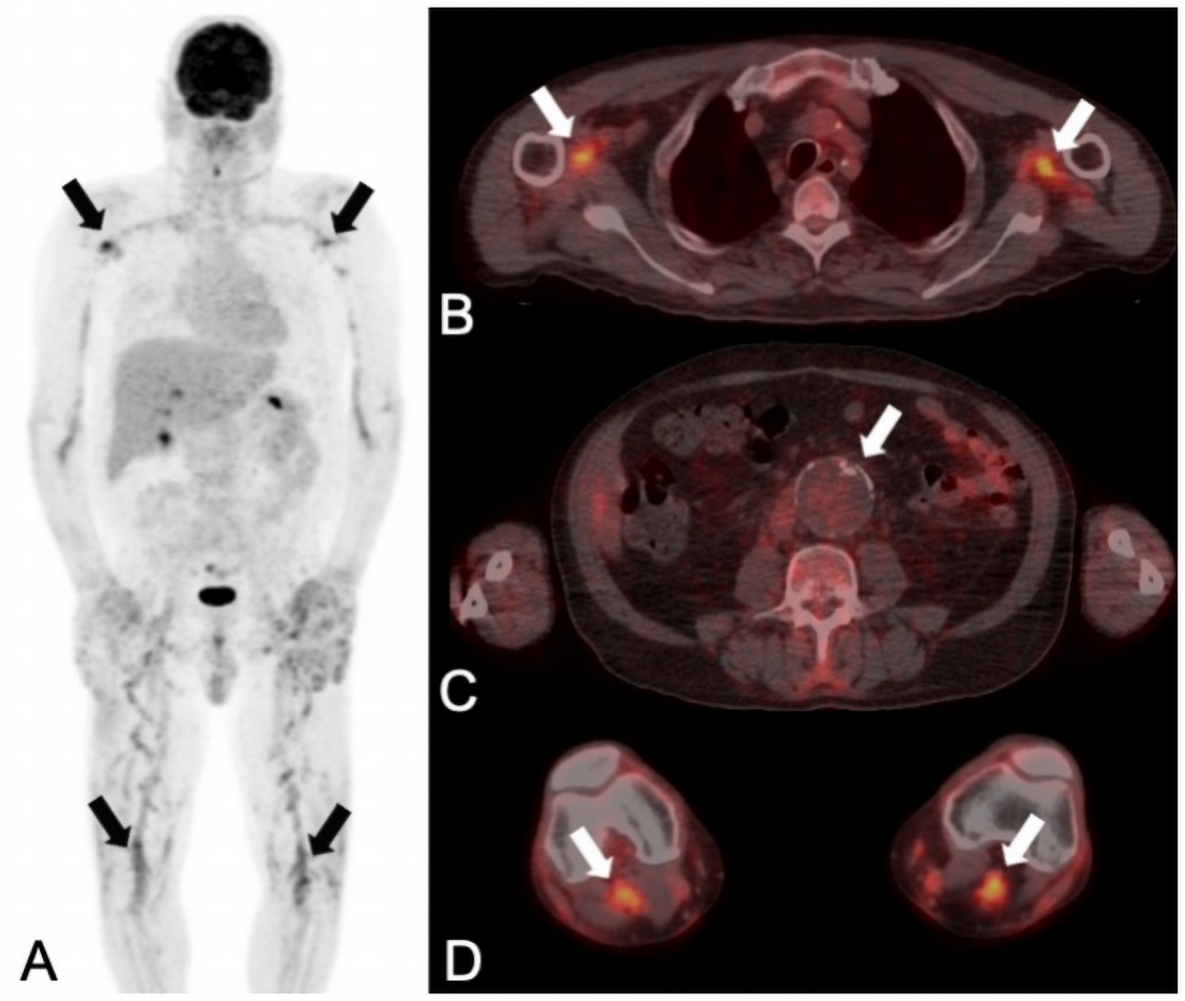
PET/CT with confirmed impact on management of an incidental finding. A 61-year old man was presented with recurrent episodes of fever and an elevated C-reactive protein level (154 mg/L). A PET/CT with the question for mycotic aortic aneurysm (MAA) or recurrence of lymphoma was acquired (A: maximum intensity reconstruction, B, C and D: fused axial PET/CT images). Lymphoma recurrence and MAA were correctly ruled out by both readers (no increased uptake in the abdominal aneurysm (white arrow in C)), as confirmed by long-term clinical follow-up. As an incidental finding with impact on patient management, PET/CT detected arteritis of the peripheral arteries (as demonstrated in the axillary (upper black arrows in A and white arrows in B) and popliteal arteries (lower black arrows in A and white arrows in D)). At the last clinical visit (826 days after PET/CT) the patient had continuous therapy with glucocorticoids. The patient was doing fine with no clinical signs of recurrent lymphoma, infection or inflammation.
